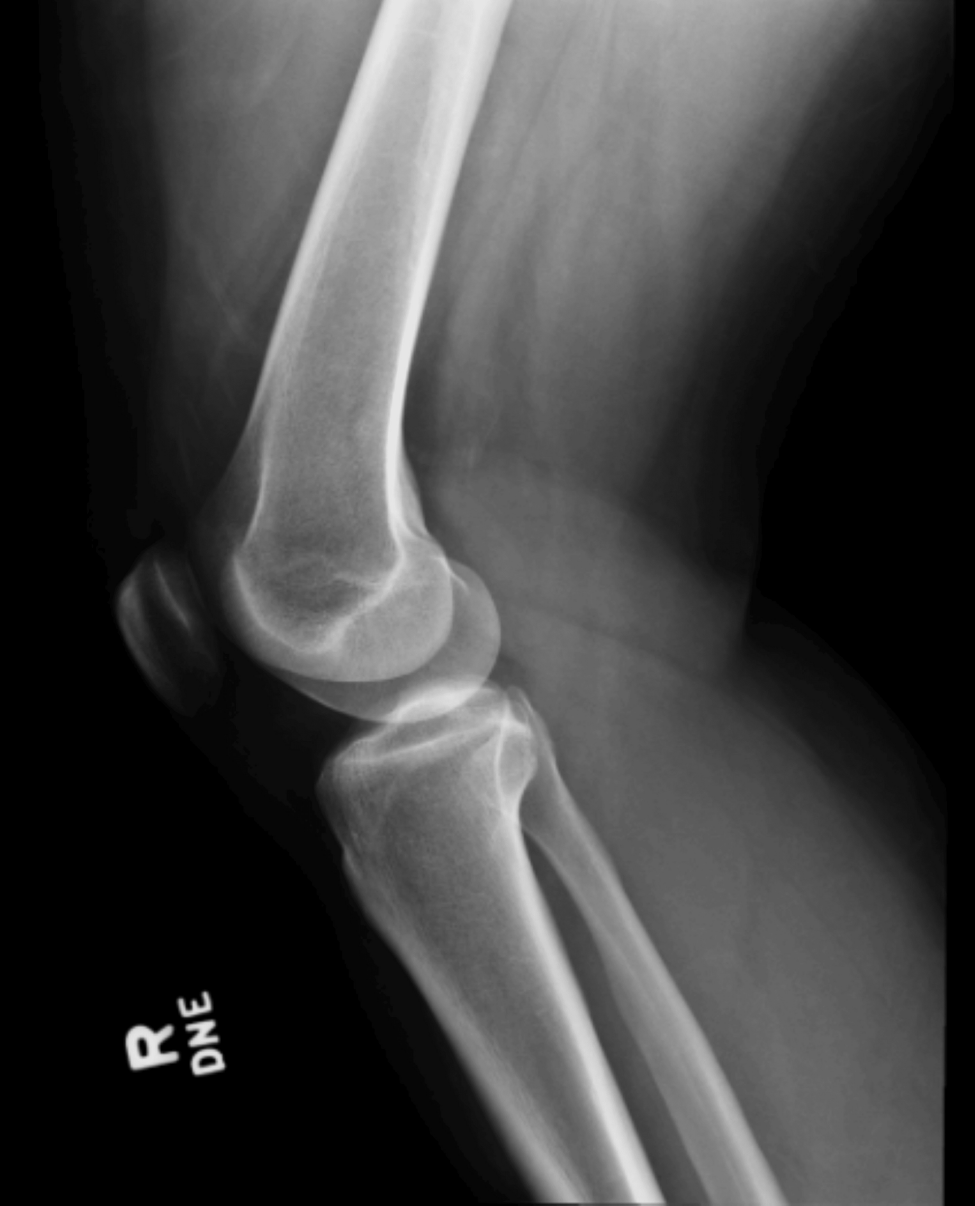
Lateral radiograph of the patient’s right knee a few hours after injury
